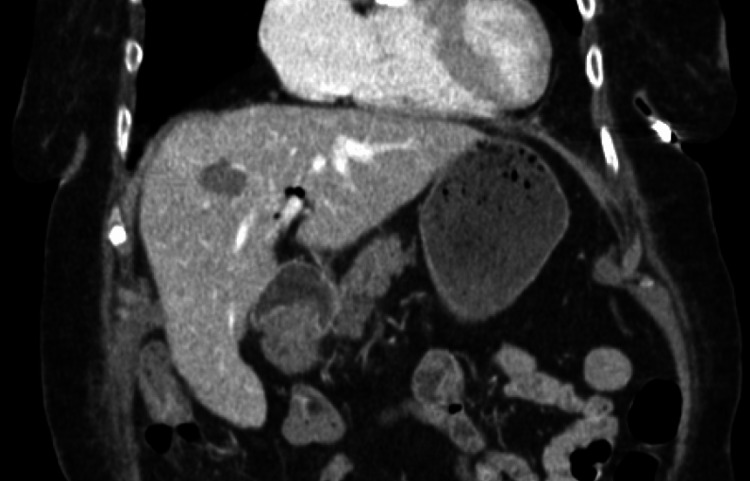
Follow-up CT after two days of tranexamic acid therapy revealing pseudoaneurysm thrombosis and stable peripancreatic hematoma
